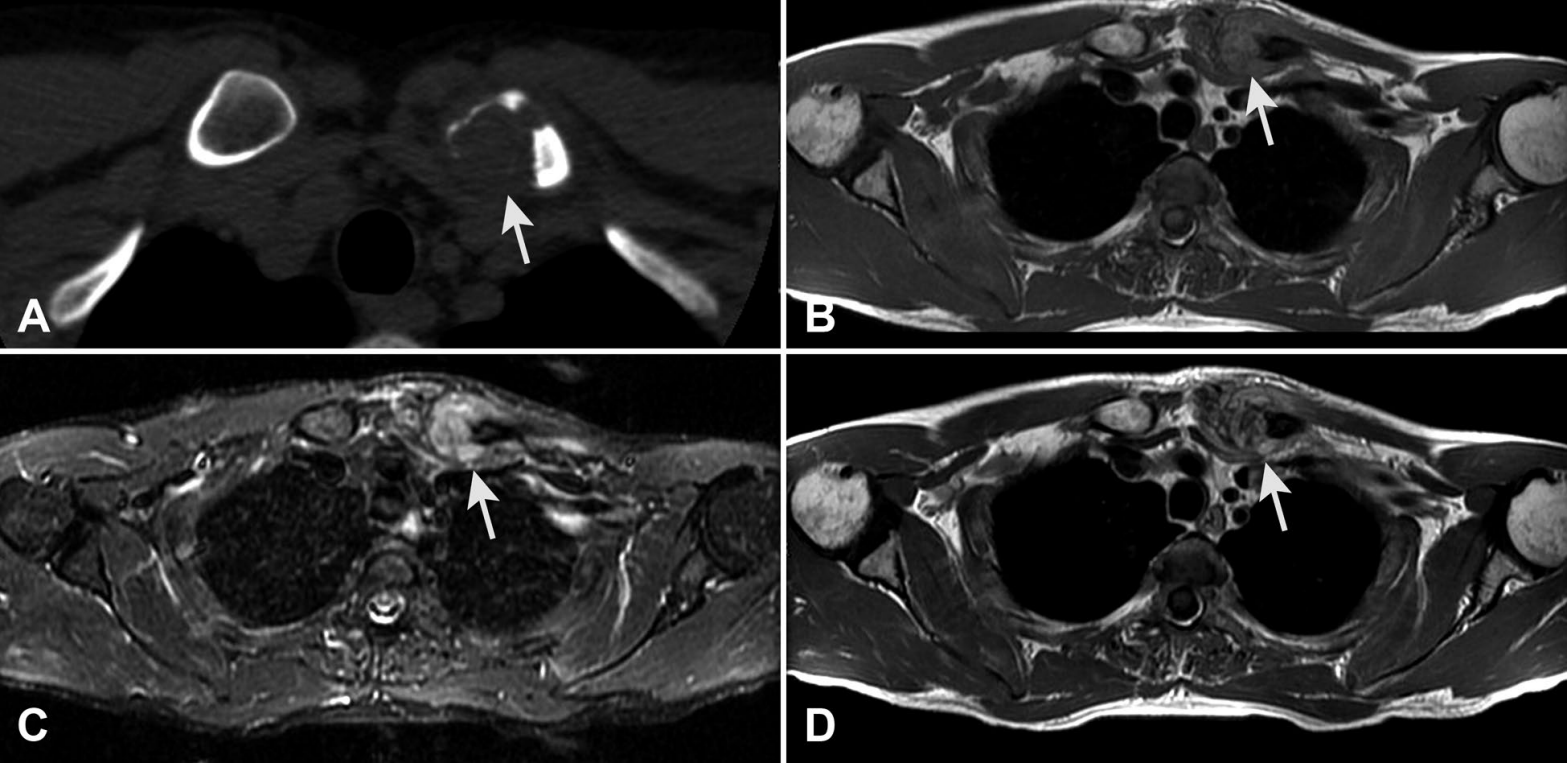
On CT A the lesion (see arrows) involves the medial end of the clavicle and is purely lytic; the cortex is destroyed and soft tissues are invaded. On axial MRI the signal is isointense on T1-weighted (B), and heterogeneously hyperintense on fat-saturated T2-weighted (C) and after contrast medium injection (D)
